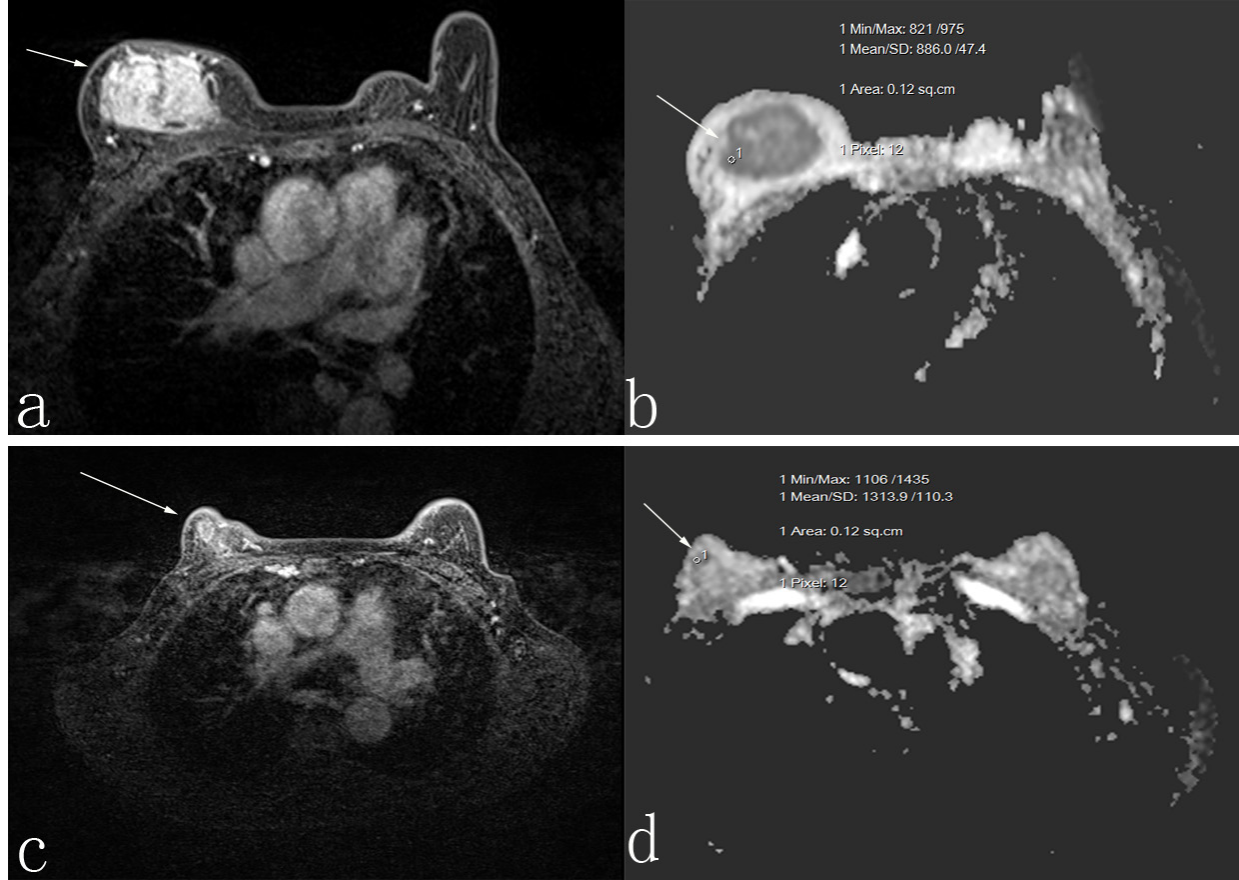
A 50-year-old woman who was responder with invasive ductal carcinoma Before neoadjuvant chemotherapy, the lesion diameter was 5.5 cm in transverse contrast-enhanced T1-weighted image a. the apparent diffusion coefficient (ADC) value was 1.118 × 10^-3^ mm^2^/s b. After neoadjuvant chemotherapy, the lesion diameter was 3.4 cm in transverse contrast-enhanced T1-weighted image c., and the ADC value was 1.30 × 10^-3^ mm^2^/s d.
